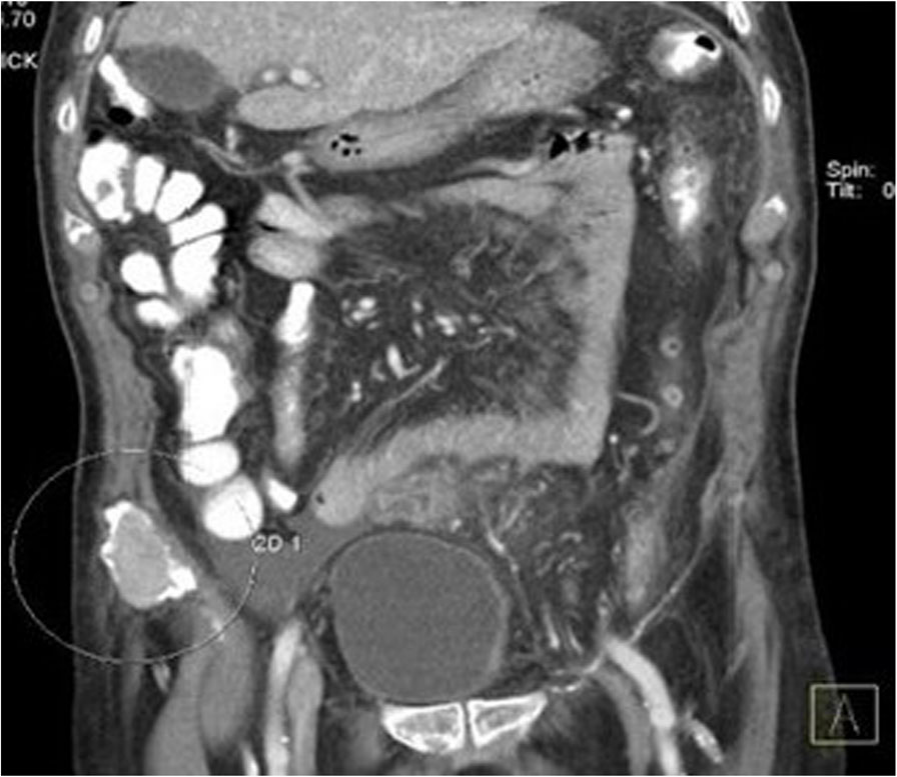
Computed tomography scan with contrast of the abdomen. Sigmoid diverticulosis and a 4.96 cm diameter right iliac crest mass, accompanied by 6.5 × 4.8 cm of fluid collection. The circle is showing the right iliac crest mass that was confirmed as a plasmacytoma
